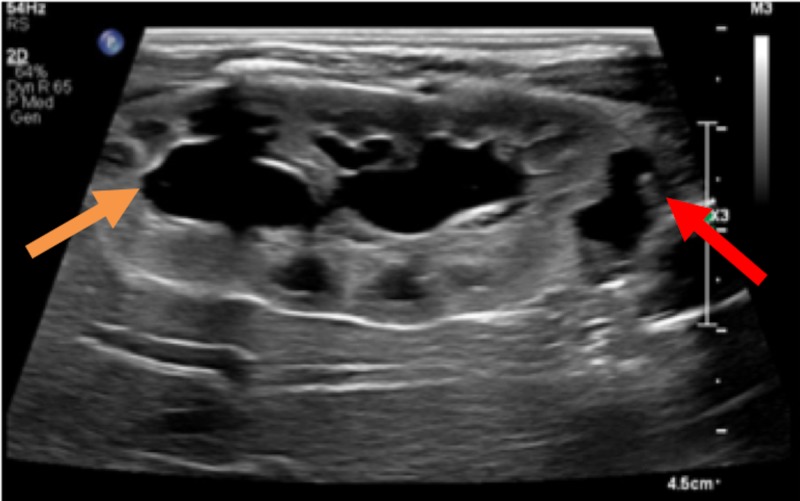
Renal ultrasound Prone ultrasound image of the right kidney in the sagittal plane demonstrates a duplicated collecting system. The orange arrow points to the upper pole collecting system and the red arrow points to the lower pole collecting system.
